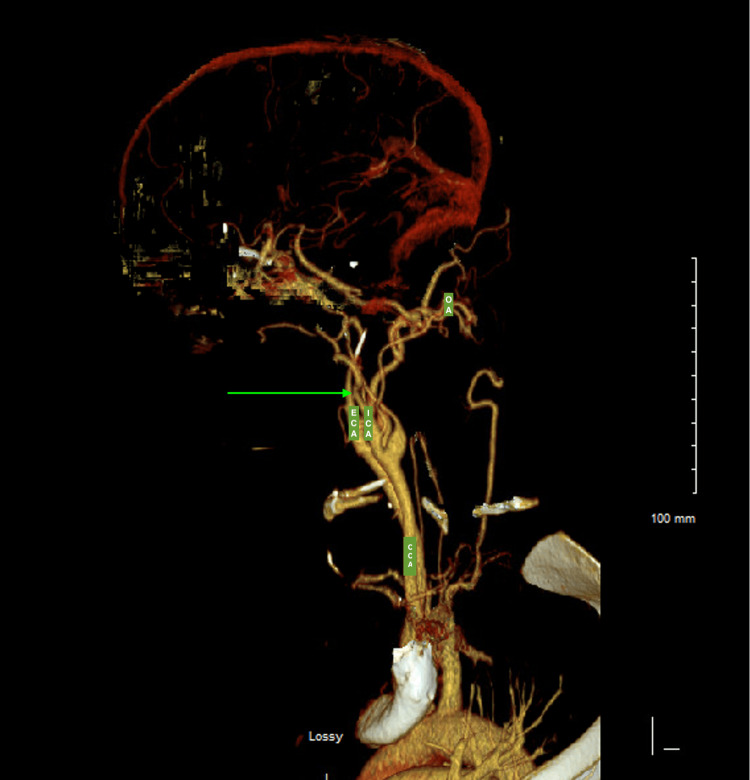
Computed tomography angiography of the head and neck with intravenous contrast Green arrow indicates left internal carotid artery (ICA) occlusion. Labels include CCA (common carotid artery), ECA (external carotid artery), ICA (internal carotid artery), and OA (occipital artery).
